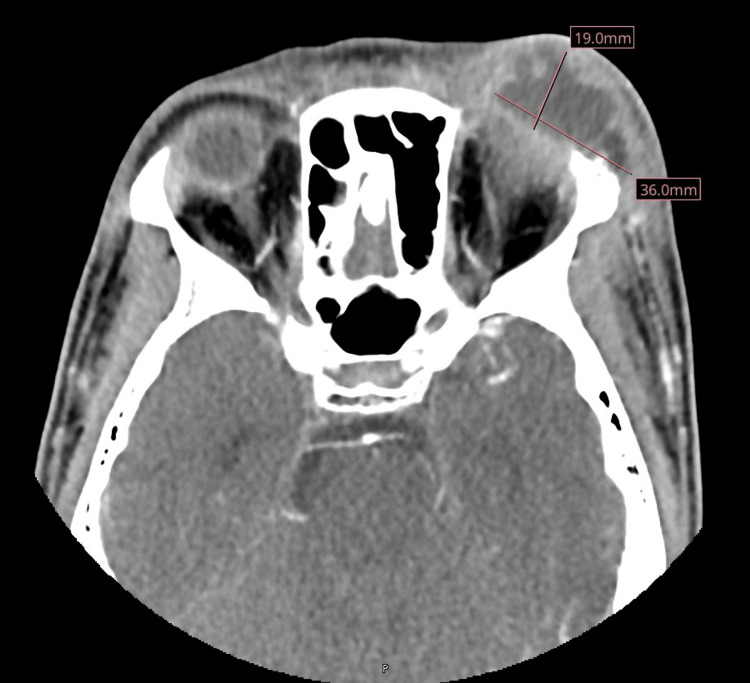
Axial CT of the orbits showing a large left superior lateral periorbital rim-enhancing abscess measuring 4.9 × 3.6 × 1.9 cm and osseous erosions of the superolateral orbital rim CT: computed tomography
